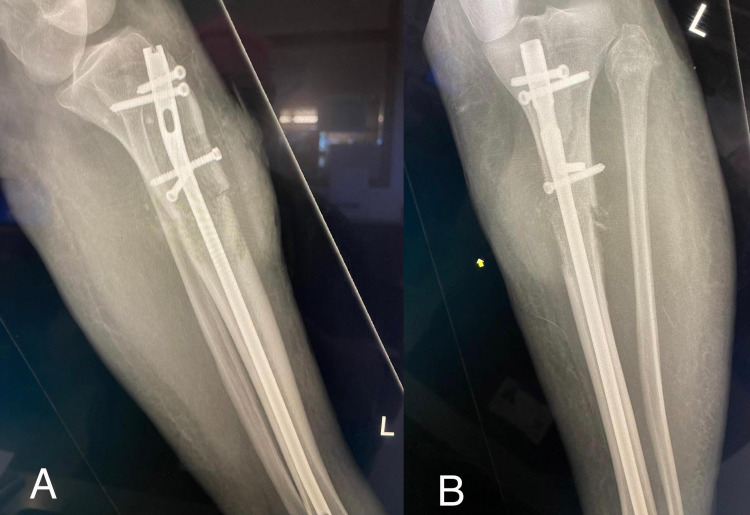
X-Ray left tibia and fibula (A-B) Lateral views of the left tibia and fibula radiography showing pathological transverse fracture of the proximal left tibial shaft post-internal fixation. The aggressive lytic lesion in the proximal left tibia appears slightly bigger as does the adjacent associated soft tissue mass with a satisfactory alignment post-internal fixation.
